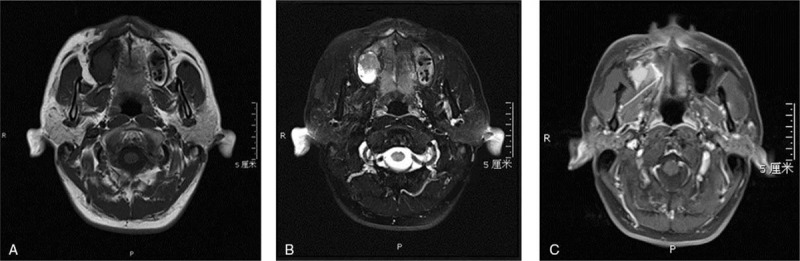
MR showed a soft tissue mass. (A) T1-weighted image (axial). (B) T2-weighted image (axial). (C) Contrast-enhanced T1-weighted image (axial).
